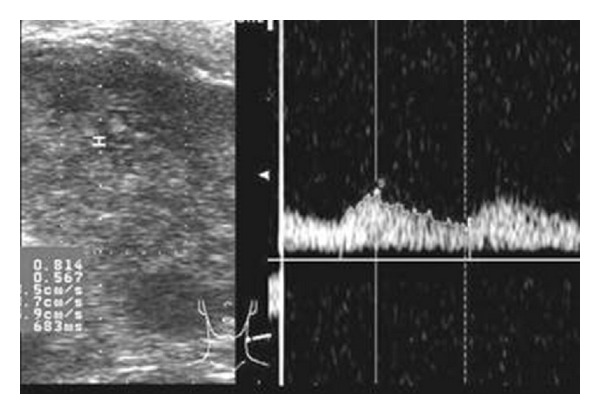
On USG neck, hypoechoic mass is seen on the left parapharyngeal space showing mild vascularity on Doppler study.
